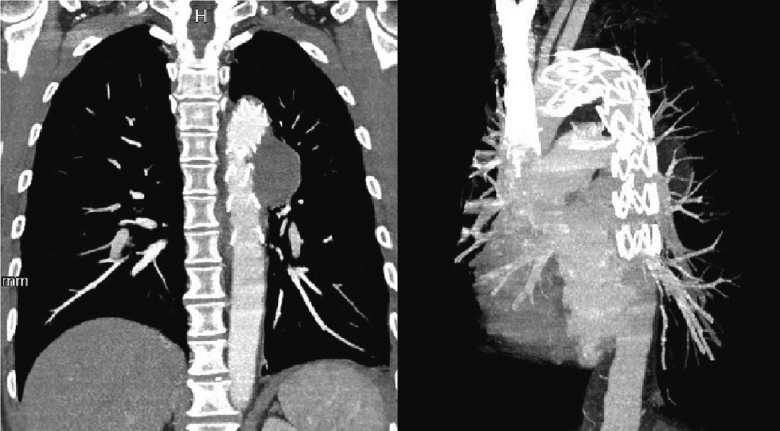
Coronal and three-dimensional computed tomography angiography (CTA) shows stent graft in the descending aorta as well as complete thrombosis of pseudoaneurysm.
